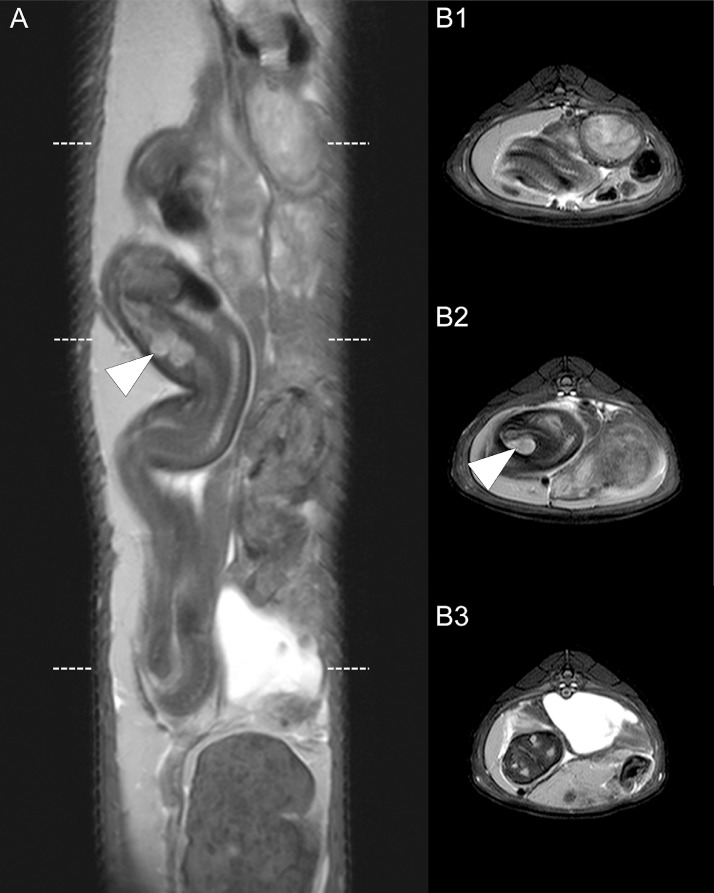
T2-weighted images of the fetus in situ in the coronal (A) and transversal (B1-3) views. The MRI examination displays the soft tissue in high resolution. The arrowhead points to the head of the fetus. The MRI examination did not detect pathologies or injuries.
